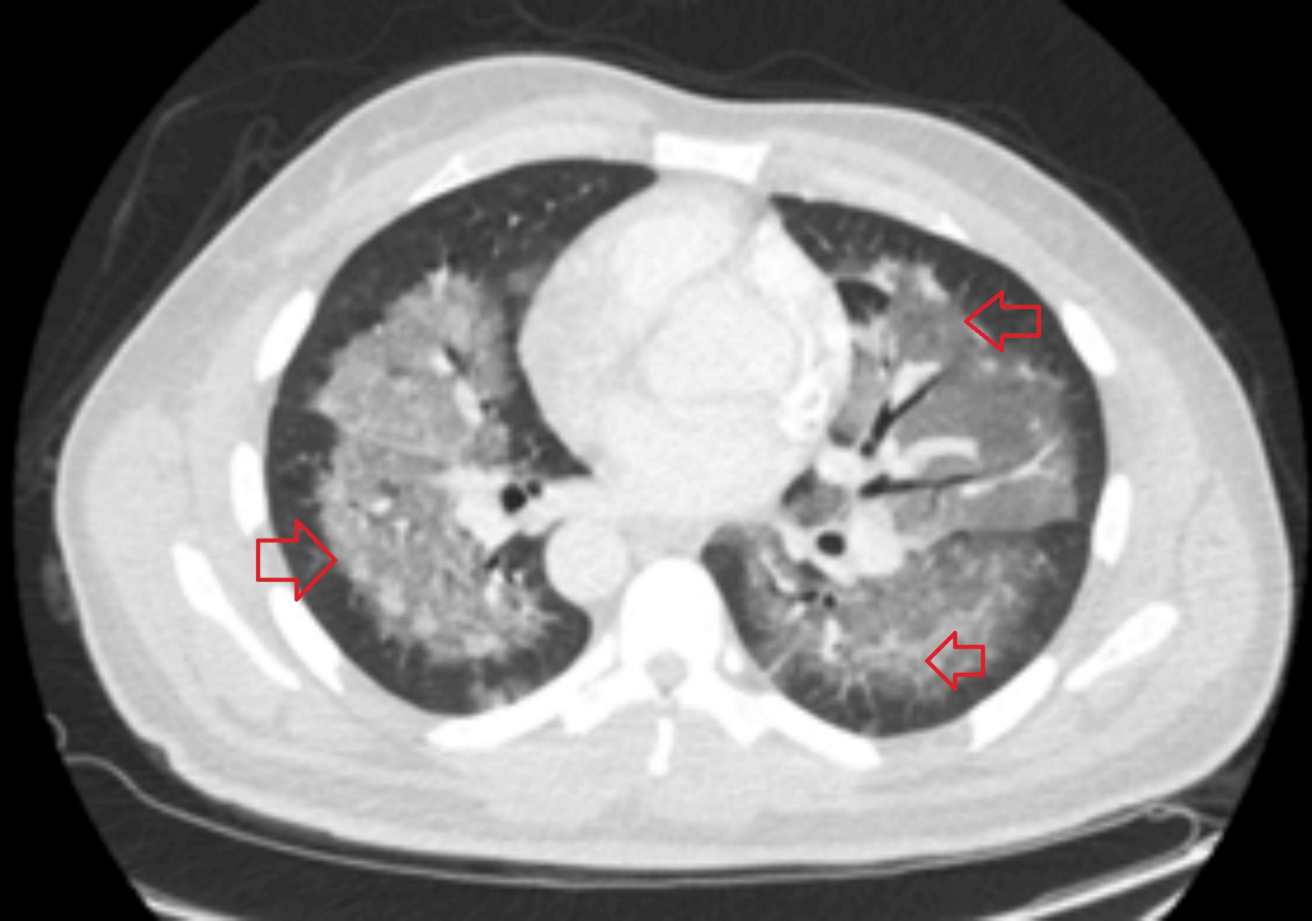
CT chest scan revealing confluent ground-glass opacities in centrilobular regions throughout both lungs.
